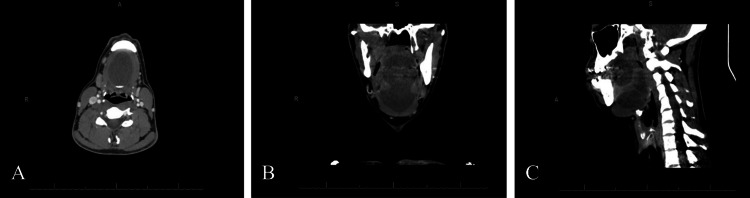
Contrast-enhanced CT neck-soft tissue with contrast of patient’s dermoid cyst at initial presentation to the emergency department. A: Axial view; B: Coronal view; C: Sagittal view
